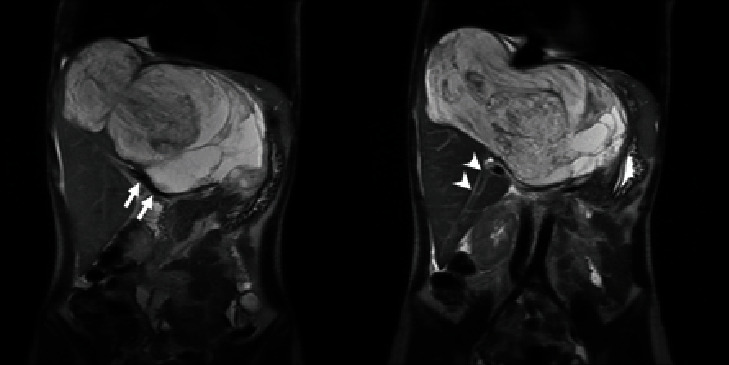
MRI T2-weighted coronal view, showing the huge heterogenous liver mass. The hepatic pedicle (arrows) and segment 6 pedicle (arrowheads) were not involved by the tumor.
